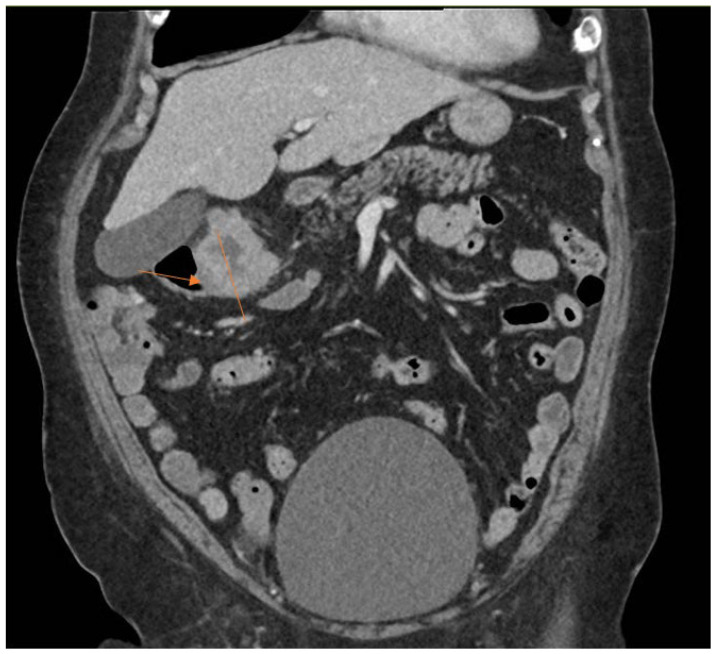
Coronal cut of CT-AP scan showing colorectal carcinoma as indicated by arrow.
